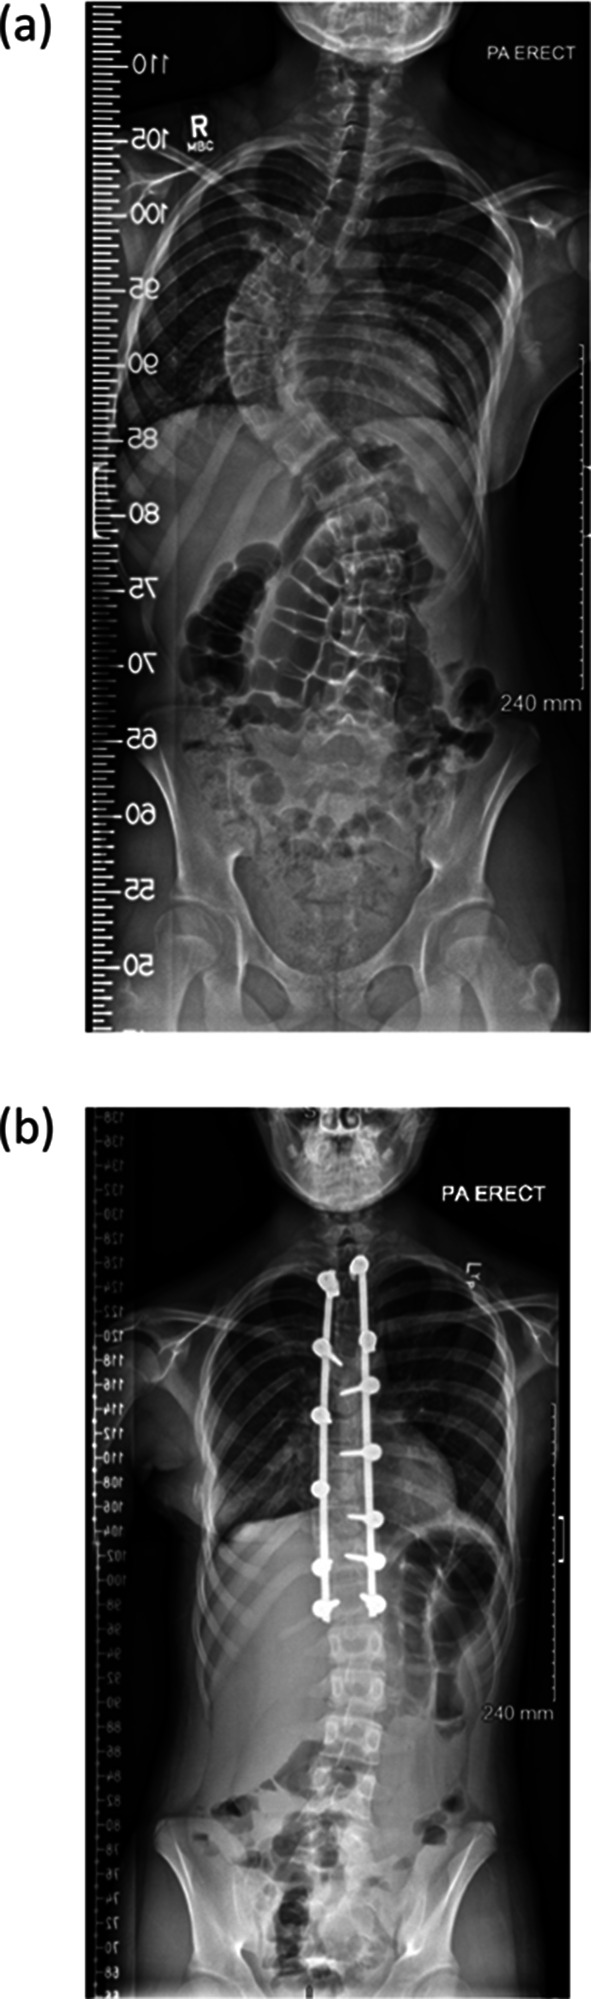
Radiographic images before and after the combined anterior release and posterior instrumented fusion. a Preoperative standing antero-posterior radiograph. b Standing antero-posterior radiograph after both stages (combined anterior release and posterior instrumented fusion)
